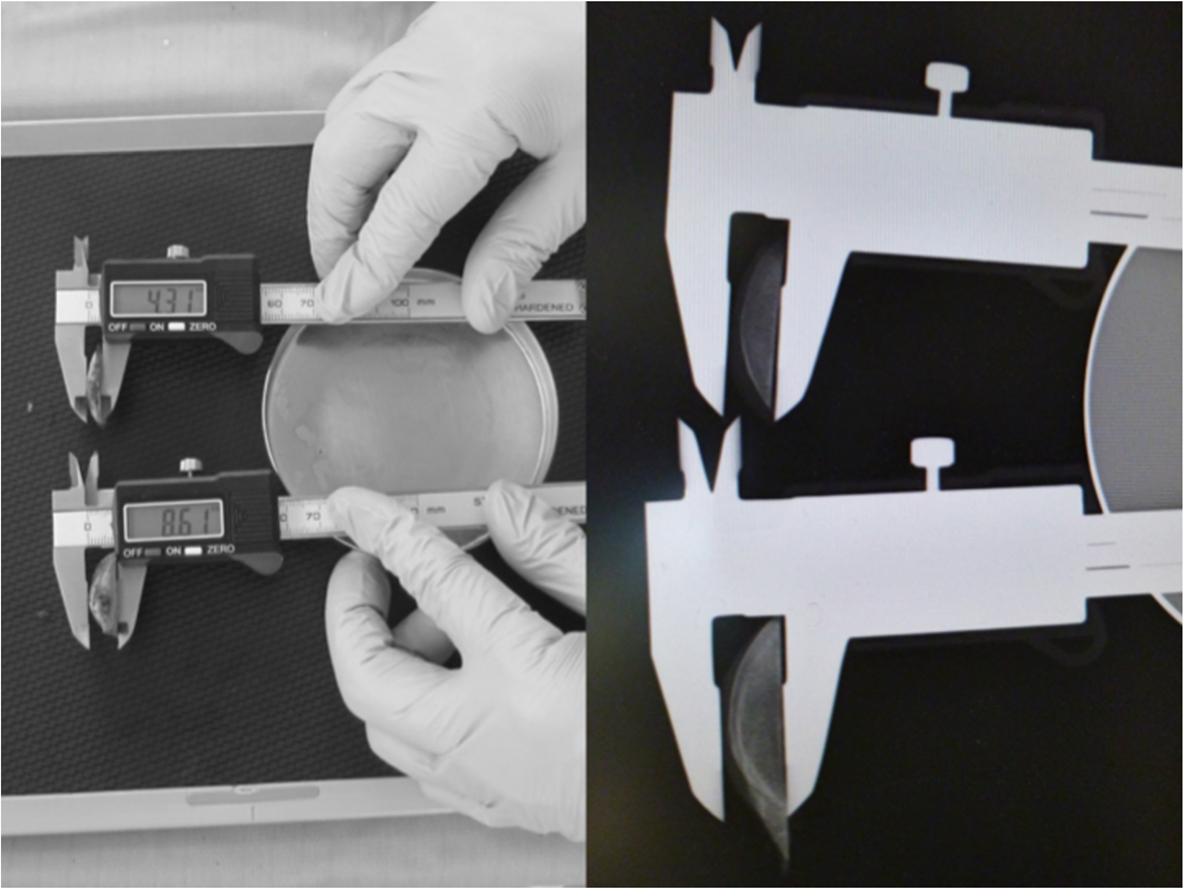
Radiographs taken parallel to the cut surface
